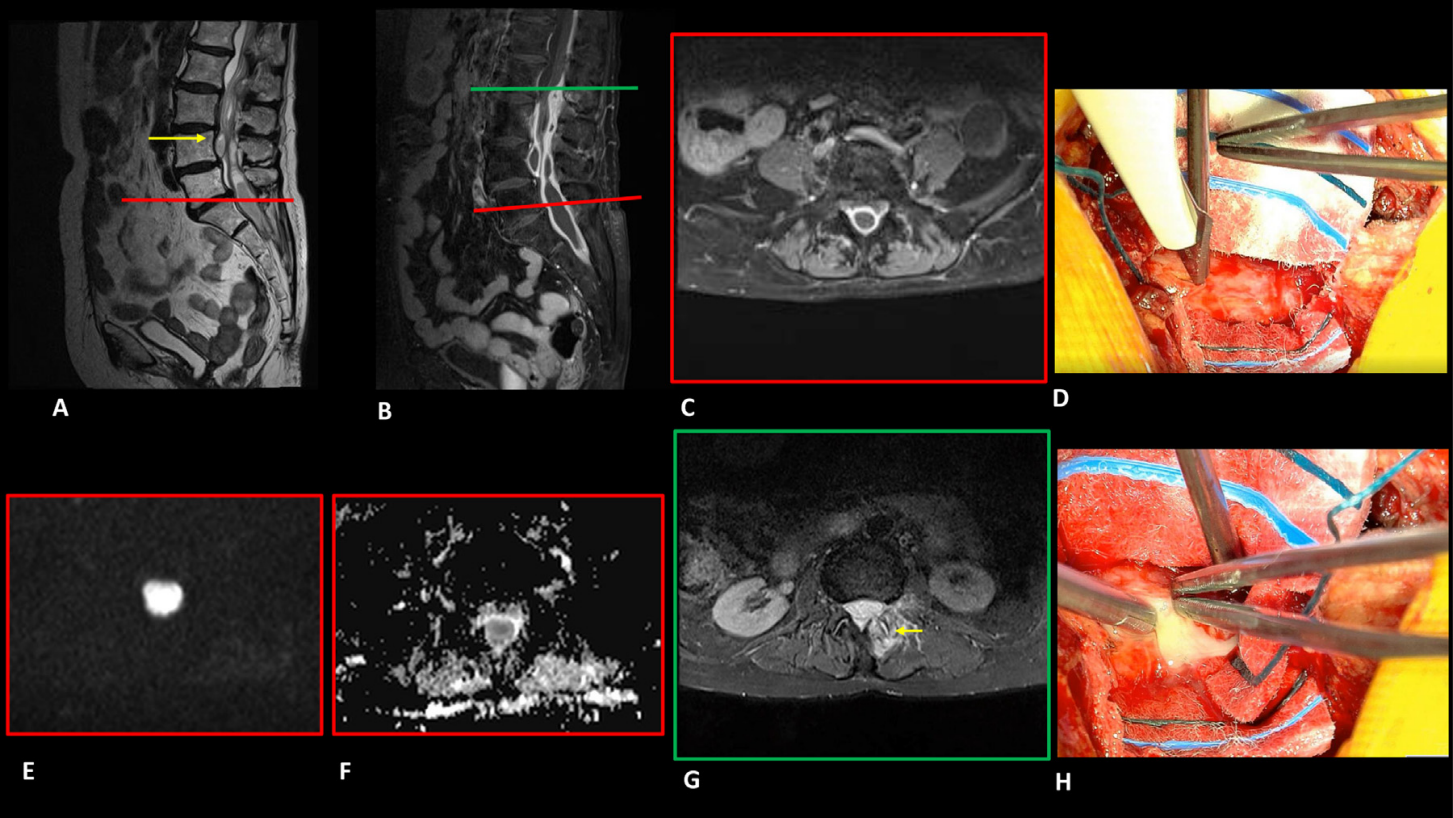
Magnetic resonance imaging of the lumbar spine. Sagittal T2 weighted MRI image (A) reveals 2 suspected intradural collections localized ventrally and dorsally to caudal roots, with hypointense margins and an internal inhomogeneous hyperintense component. Postcontrast sagittal (B) and axial (C) T1 weighted image showing intense peripheral postcontrast enhancement and internal colliquative appearance of the suspected intradural collections at L2-S2 level. Axial DWI(E)/ADC(F) demonstrating restriction within the mass. Axial (G) STIR sequence revealing pathological hypersignal of the left facet joint at level L2-L3 extended to the lamina and spinous process of L2 as well as to the musculature of the contiguous paravertebral lodge. Intraoperative photographs (D–H), (D) A L5-S1 laminectomy was performed. The epidural space and the dural surface appeared undamaged, proving the absence of epidural collections. A vertical durotomy was performed, which showed an intact fibrous capsule. (H) Yellowish purulent material oozing out after incision of the fibrous capsule, confirming the intradural location of the abscess.
